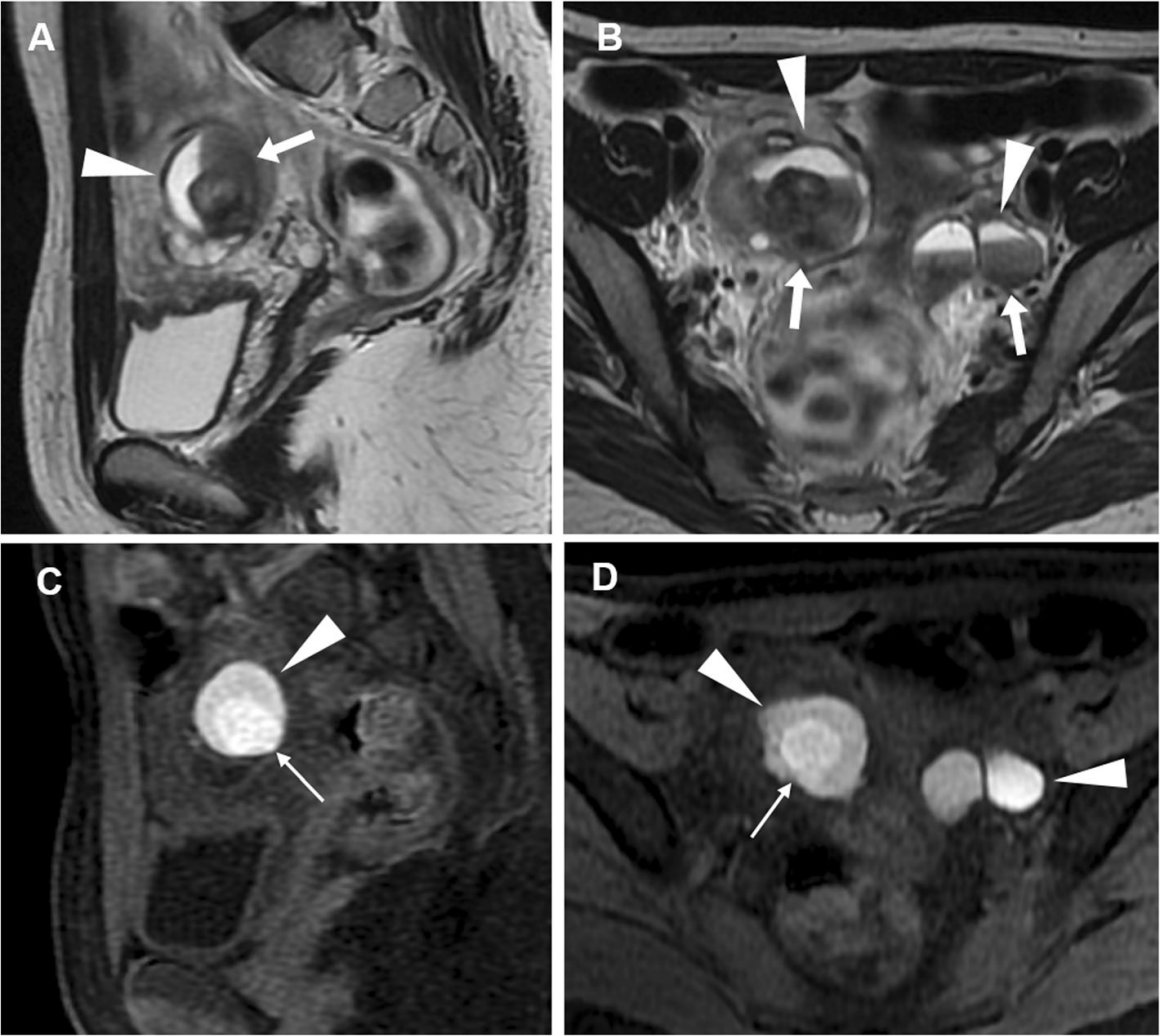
MRI of bilateral endometriomas in a 27-year-old woman with pelvic pain exacerbated in the last few days. Sagittal (a) and oblique-coronal (b) T2-weighted images show bilateral endometriomas with fluid-blood levels (arrowheads) and low signal intensity in the declivous portion of the cyst (‘shading sign’, arrows). On sagittal (c) and oblique-coronal (d) precontrast fat-suppressed T1-weighted images, the cysts demonstrate high signal intensity (arrowheads). Note the clot within right endometrioma with very high T1 signal intensity (thin arrows)
